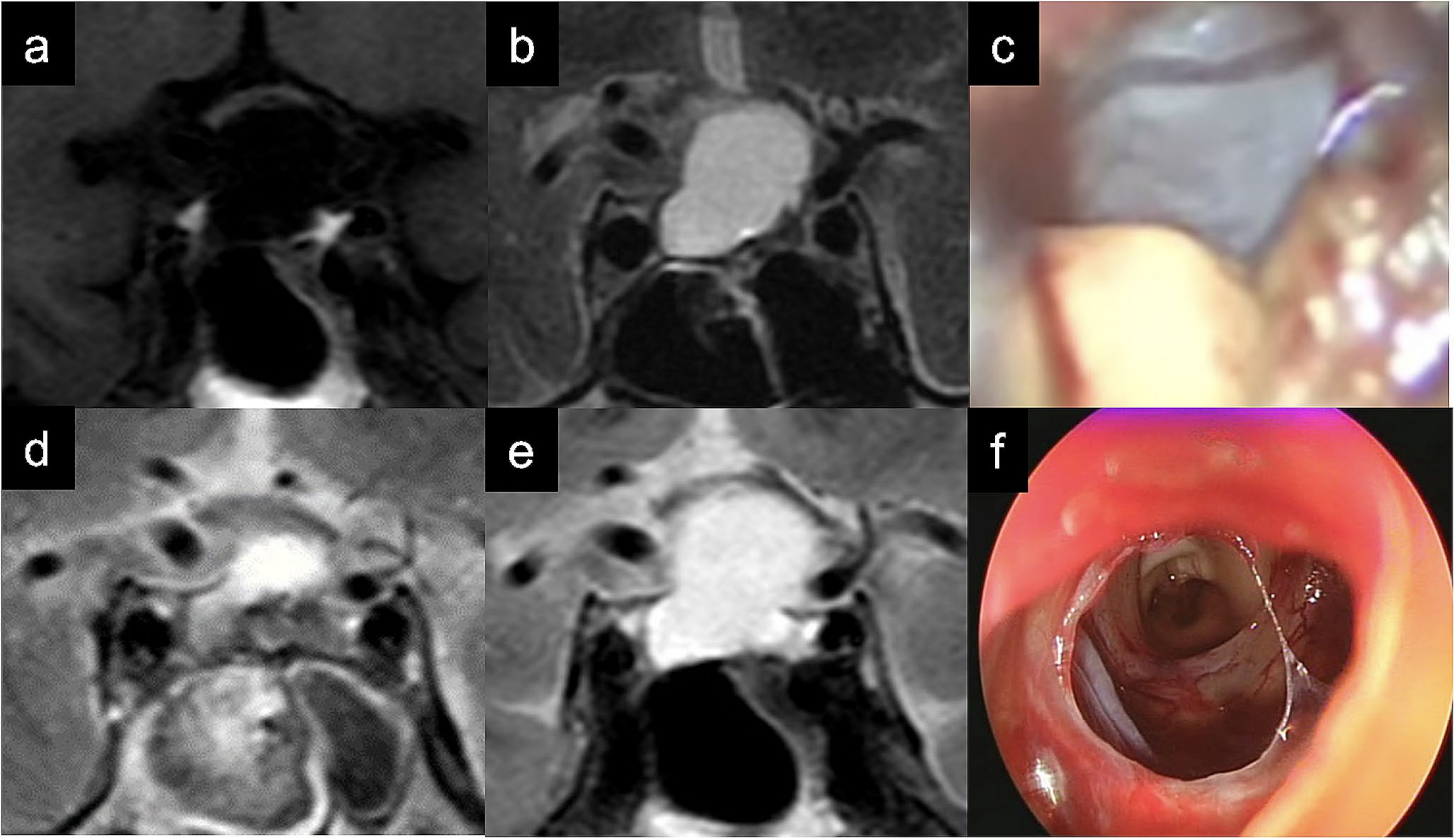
Representative case for type C Rathke’s cleft cyst (Patient No. 32). (a) T1-weighted image (T1WI) showing low intensity in the cyst; (b) T2-weighted image (T2WI) showing high intensity in the cyst; (c) Intraoperative findings showing the thin cyst wall, and when it was cut, clear, watery content flowed out; (d) T2WI obtained 1 week postoperatively showing that the cyst is shrinking; (e) T2WI obtained 3 months postoperatively showing that the cyst had reaccumulated and the optic nerve is compressed; (f) Intraoperative findings at the time of cyst recurrence showing that the cyst and cyst wall were opened and the optic nerve is observed under endoscope
